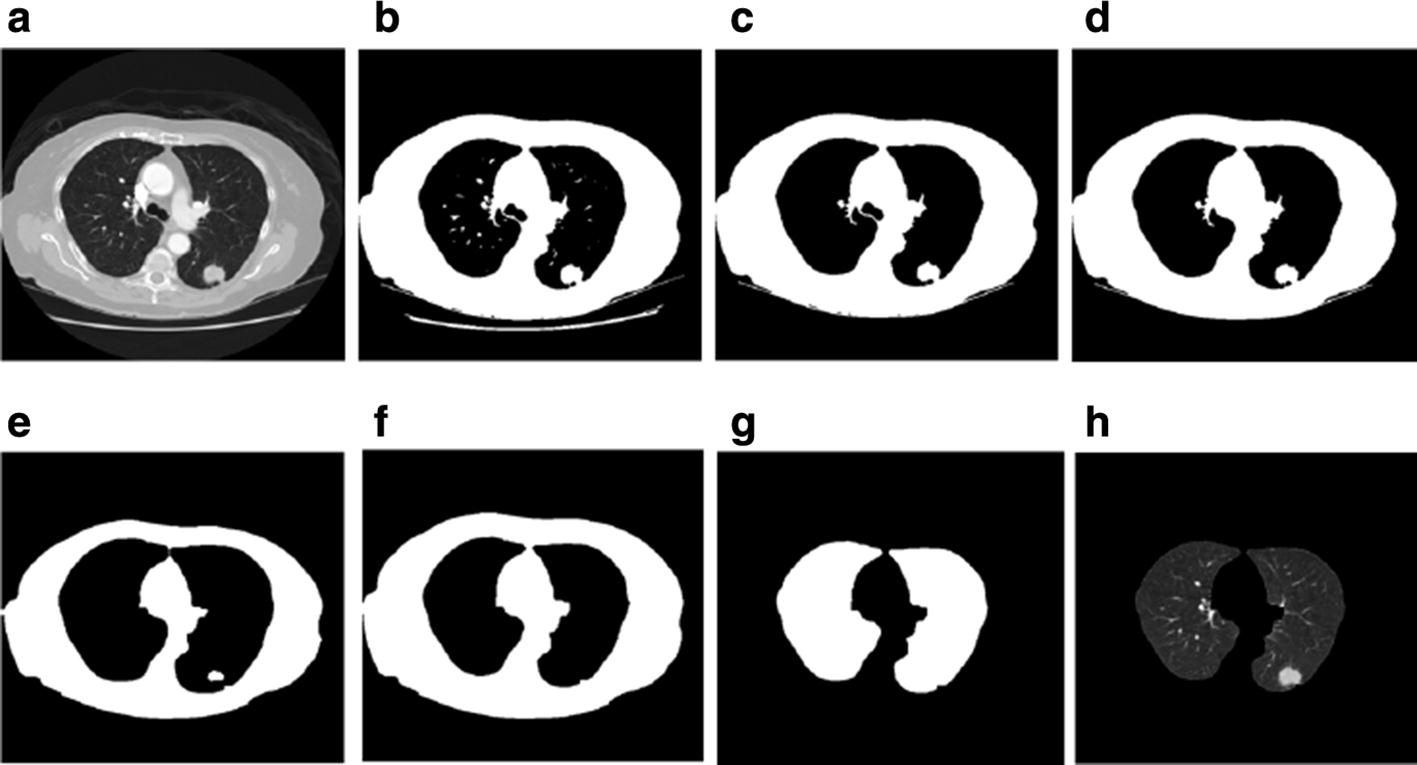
Steps of pulmonary parenchyma extraction. a Original CT image; b binarization of the CT image; c preliminary extraction of pulmonary contour; d filling airways; e contours corrosion; f contour mending and expansion; g pulmonary masking; h pulmonary parenchyma image
